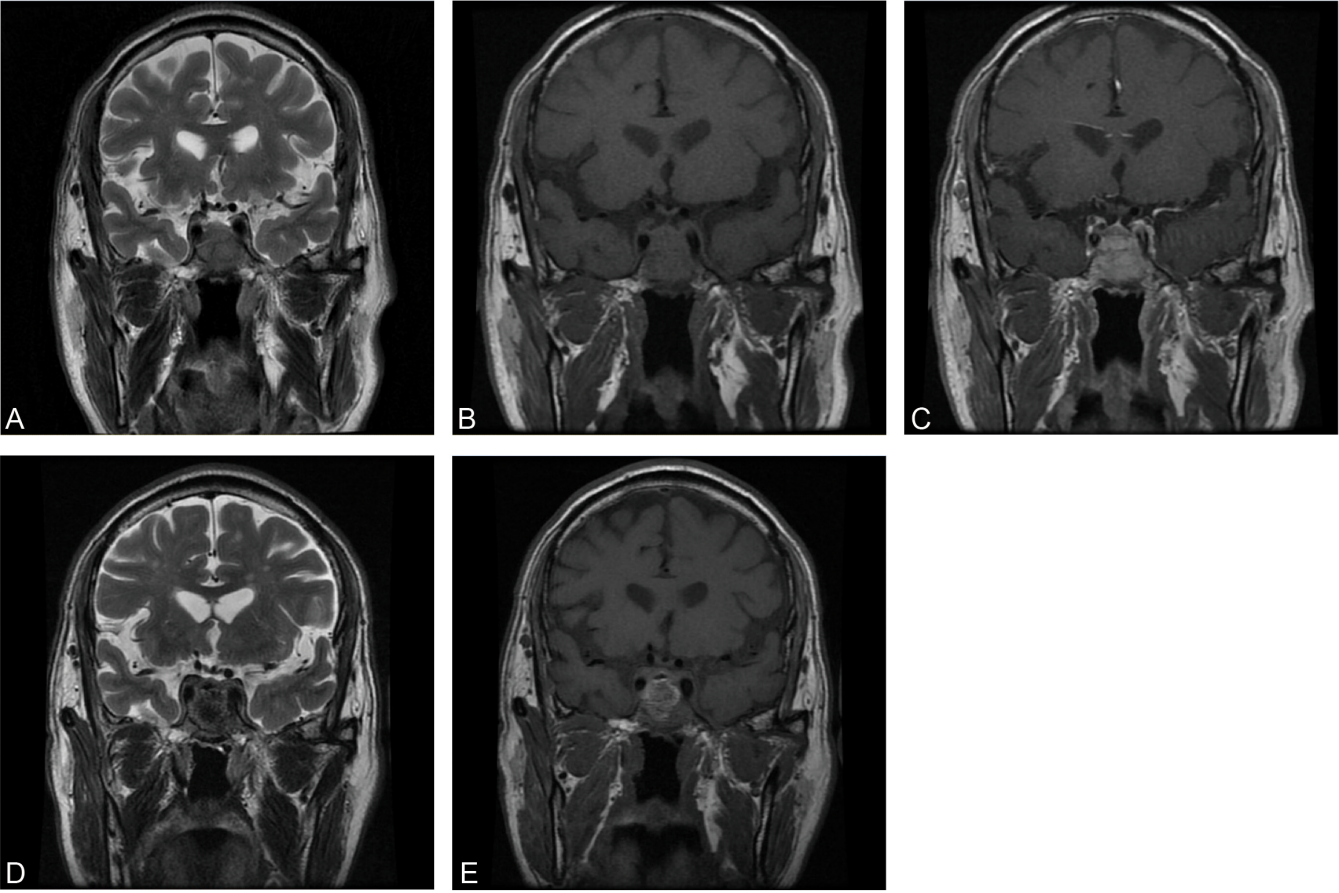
Preoperative coronal MRI of precontrast T2WI (A), precontrast T1WI (B), and enhanced T1WI (C). Postoperative coronal MRI of precontrast T2WI (D) and precontrast T1WI (E).
